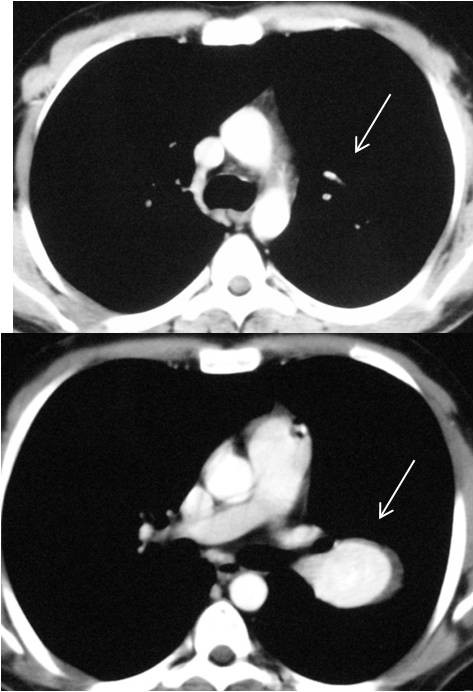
Thorax CT scans showing that in situ thrombosis in PAAs was completely resolved with immunsuppressive treatment (white arrows).
